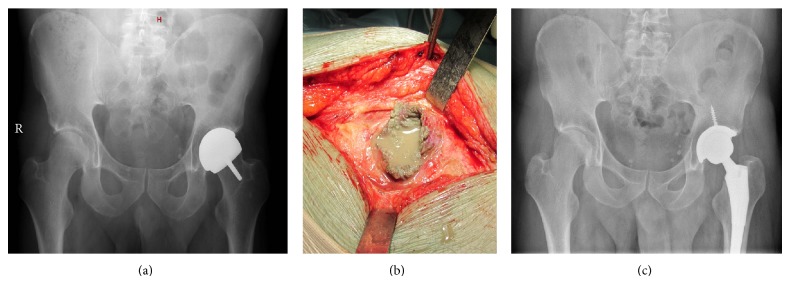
(a) Anterior-posterior radiograph of the pelvis prior to revision surgery, showing no relevant radiological findings related to the implant; (b) intraoperative appearance of the pseudotumour during revision surgery; and (c) radiograph of the same patient 6 months after revision.
